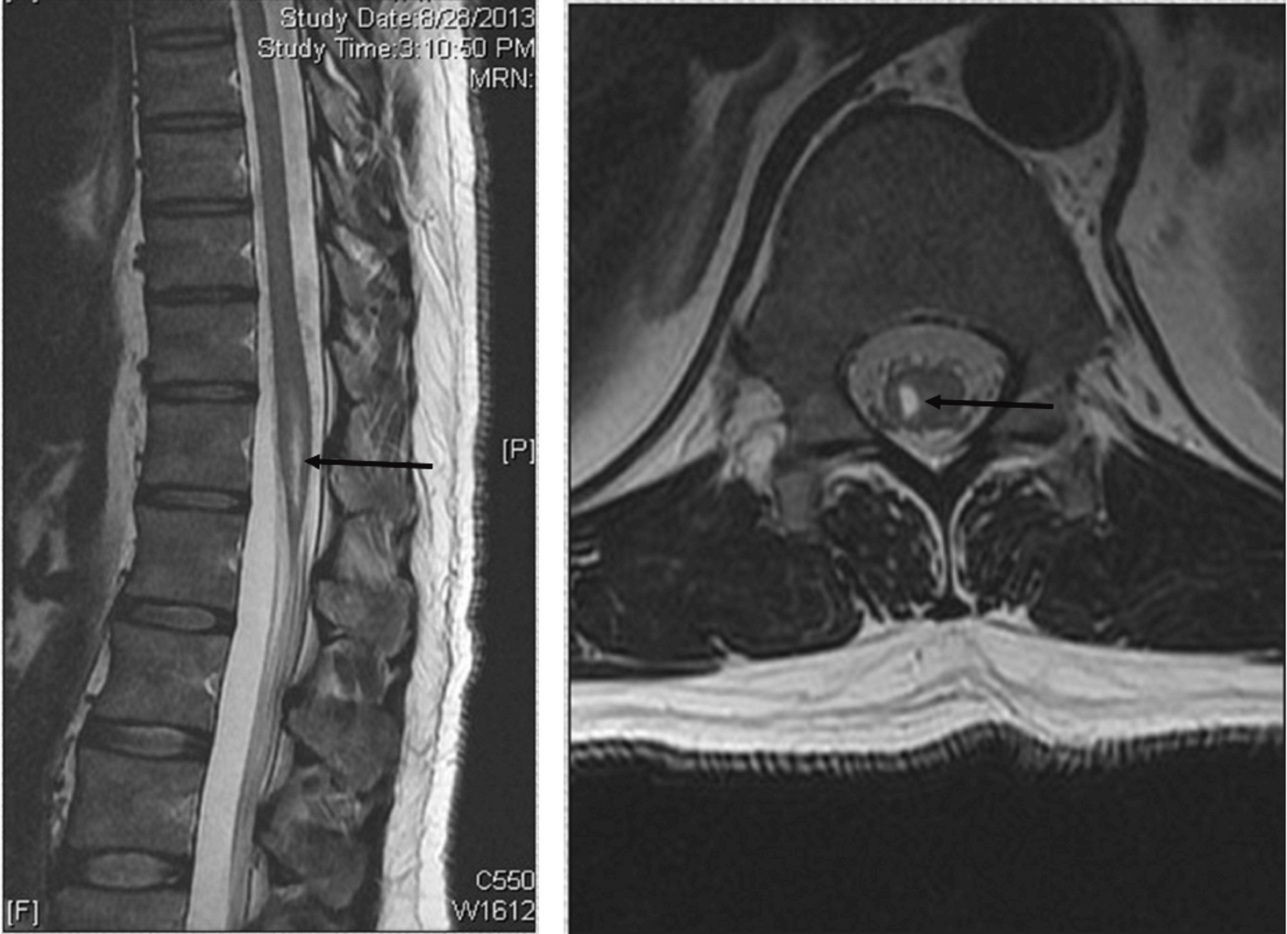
MRI: Sagittal T2-weighted (left) and axial T2-weighted (right) images of the lumbosacral spine demonstrate a lesion in the spinal cord at the level of the conus medullaris, extending from the L1 to L2 levels.
